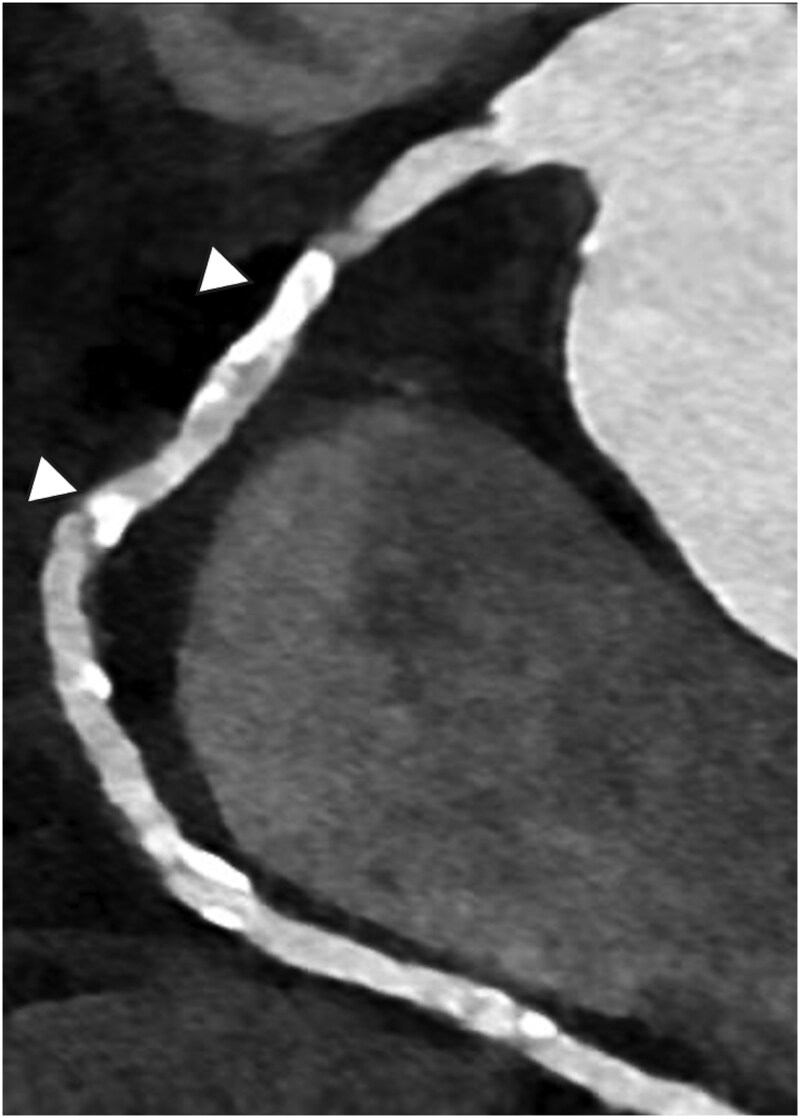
The limitations of the FLASH protocol with severe coronary calcification. A severe luminal stenosis (arrowhead) on CT was actually mild/moderate on invasive angiogram.
